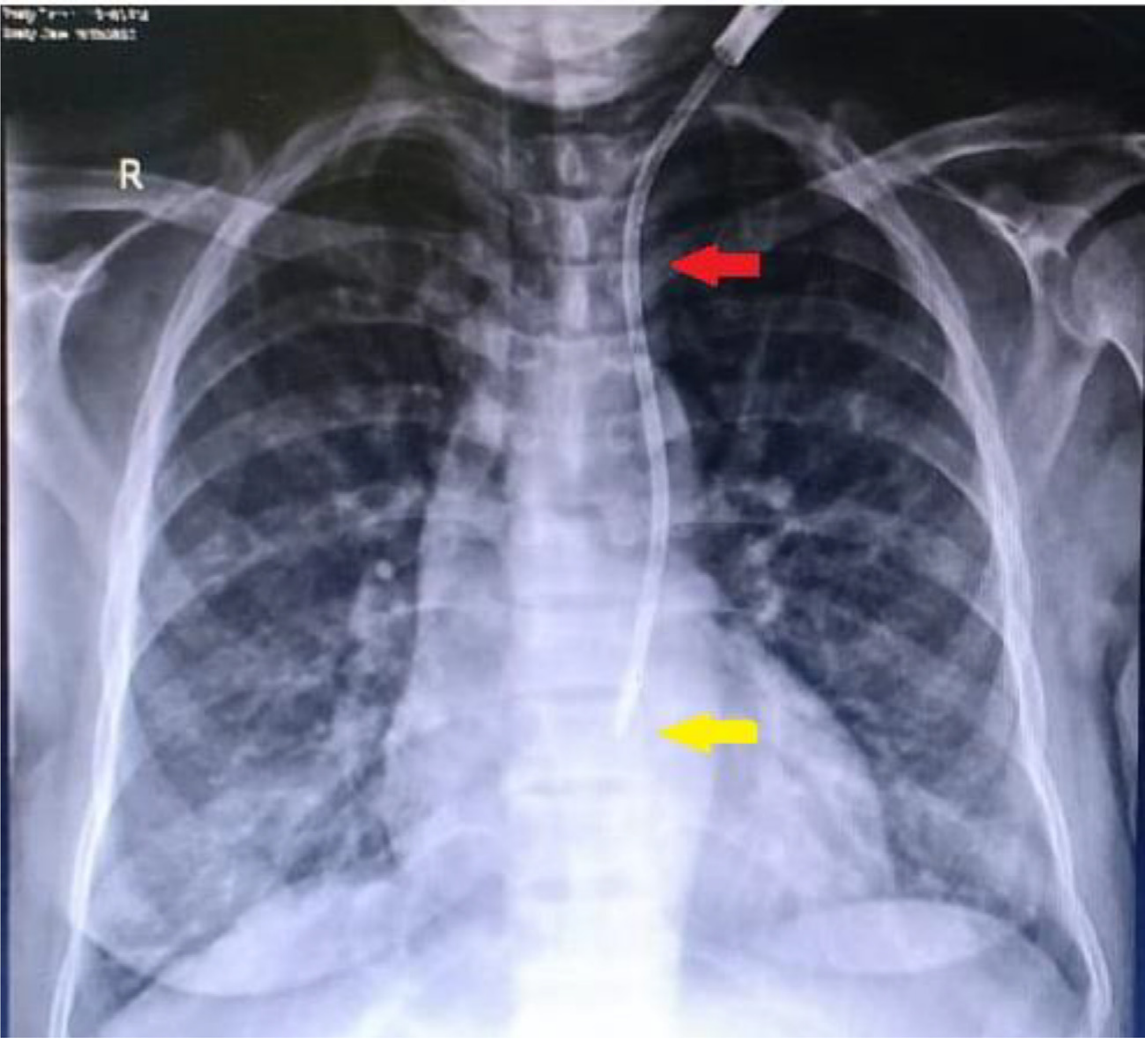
Posterior to anterior (PA) view of post-CVC insertion chest X-ray (Red arrow pointing at the lumen of CVC, Yellow arrow pointing at the tip of CVC).
